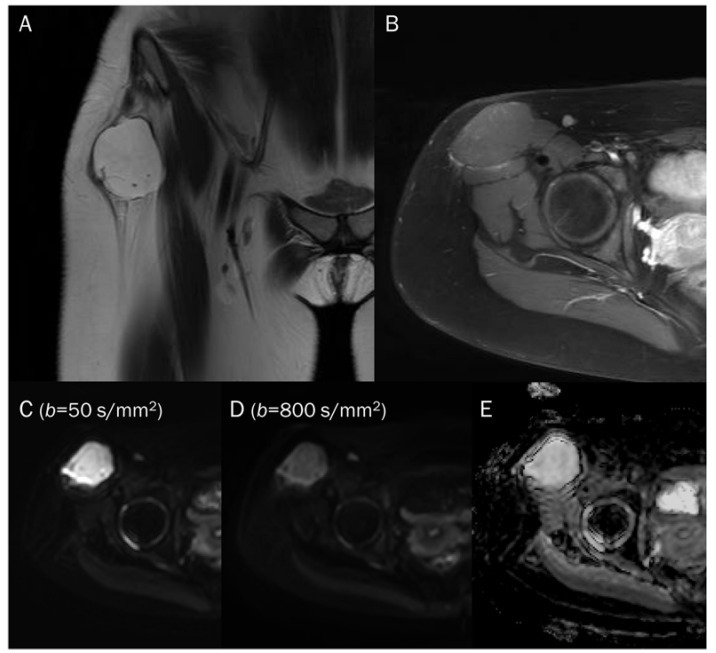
A 15-year-old female with intramuscular myxoma in the sartorius. (A) Coronal T2-weighted image shows a lobulated mass with T2 high signal intensity in the sartorius muscle. (B) Axial T1-weighted enhanced image shows peripheral and septal enhancement in the mass. (C,D) DWI with *b*-values of 50 and 800 s/mm^2^ exhibit progressive signal loss of the tumor with (E) no reduction in the ADC map, suggesting “free” diffusivity.
